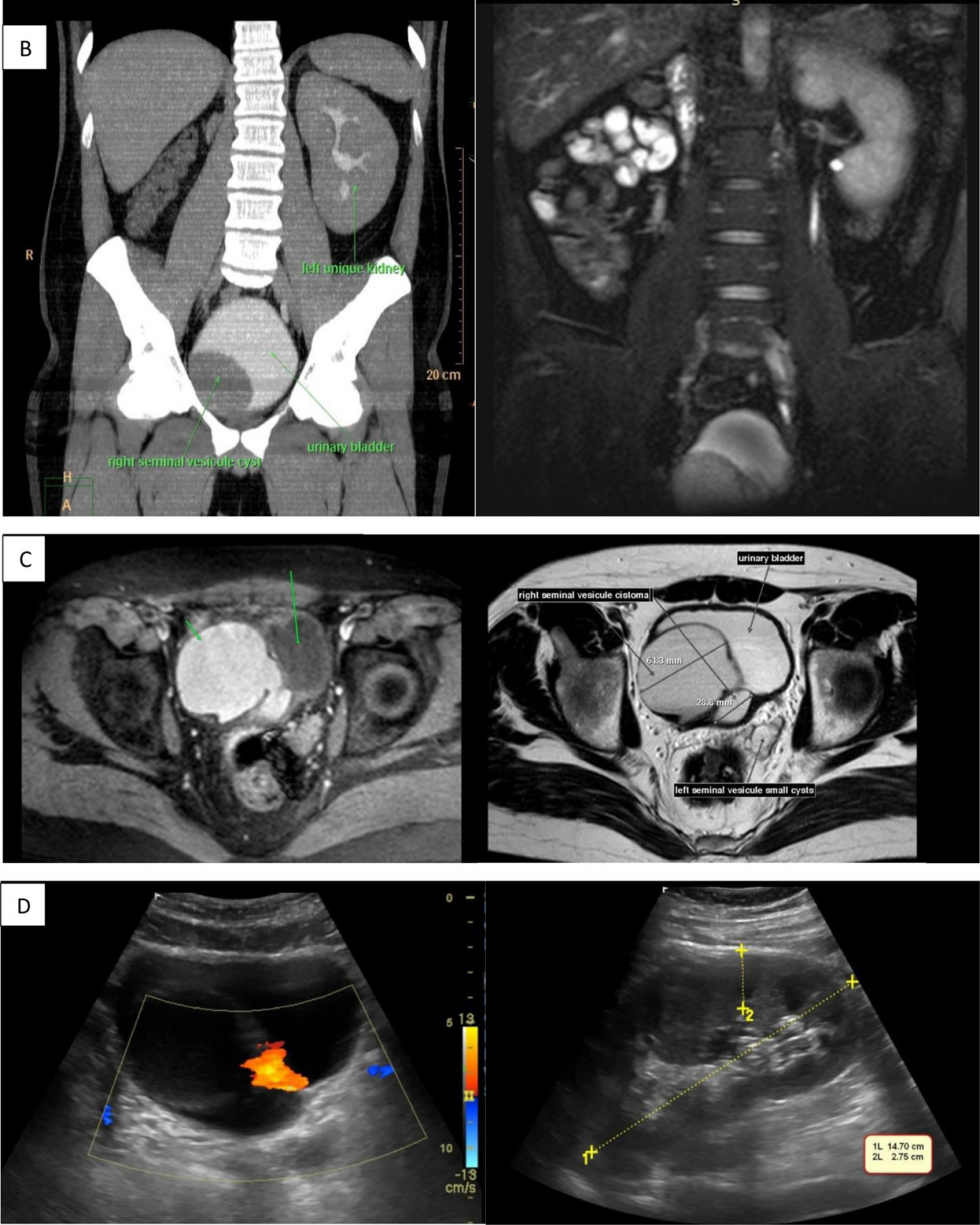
Computed tomography images (A) 3D CT: defect in the urinary bladder from right seminal vesicle cystoma and left unique kidney. (B) Coronal CT: notes the filling of the urinary bladder with contrast media and compression from the right seminal vesicle cystoma. (C) Axial CT: notes the filling of the urinary bladder with contrast media and compression from the right seminal vesicle cystoma. (D) Right cystoma prominence in the urinary bladder and a unique left urinary jet is revealed. Hypertrophied left kidney is noted.
